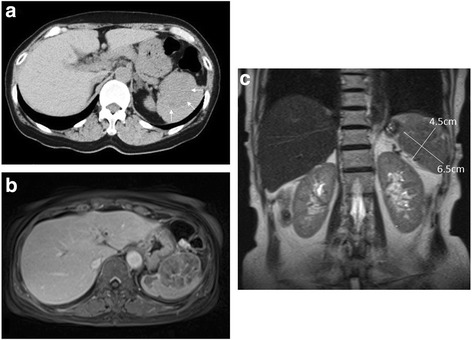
Computed tomography and magnetic resonance imaging examination. a Computed tomography performed in December 2016 shows a pale homogenous tumor in the spleen. b Enhanced magnetic resonance imaging performed in January 2017 shows a tumor with internal heterogeneity and irregular margin in the spleen. The capsule of the spleen was intact. c Coronal magnetic resonance imaging view shows a 6.5 × 4.5 cm tumor localized in the spleen. *White arrows* indicate a tumor in the spleen
